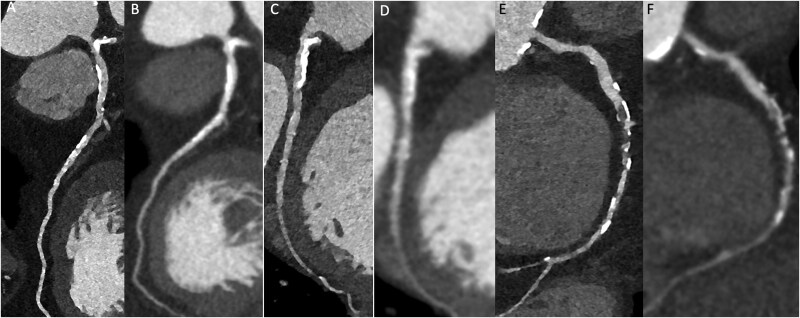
PCD-CT (Panels A, C, and E) vs EID coronary CTA (Panels B, D, and F) in the same patient 1 week earlier. Note the higher spatial resolution and superior plaque differentiation on PCD-CT compared to EID-CT.
